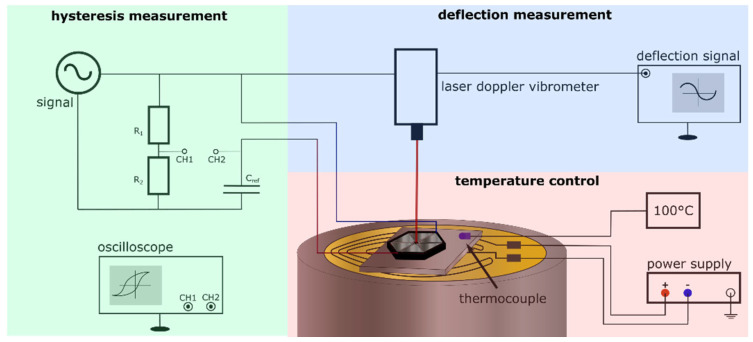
Setup for measuring the polarisation versus the electric field hysteresis loop of the piezoelectric device with a Sawyer−Tower circuit (green). As a measure of performance, the deflection of the MEMS was measured by a laser doppler vibrometer (blue). To obtain the temperature stability of the used devices, a hotplate was heated up by a DC power supply, and the temperature monitored by a thermocouple (red). Micropositioners are not shown for visibility.
